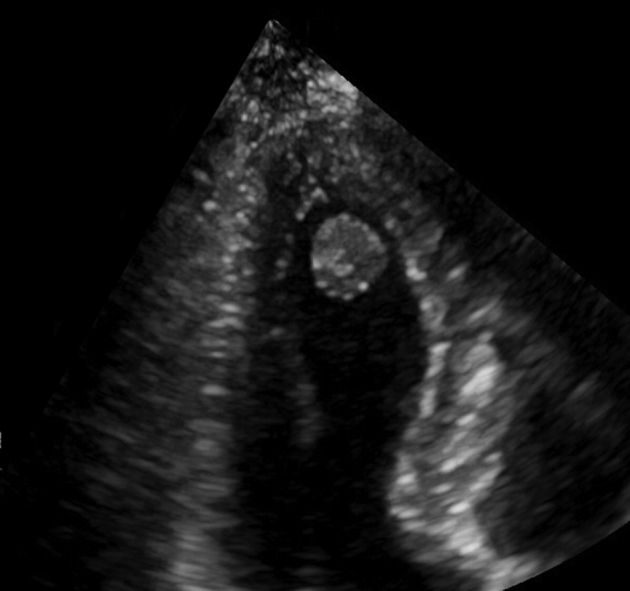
Transthoracic echocardiography of a 13-mm rounded tumor at the left ventricular apex.
